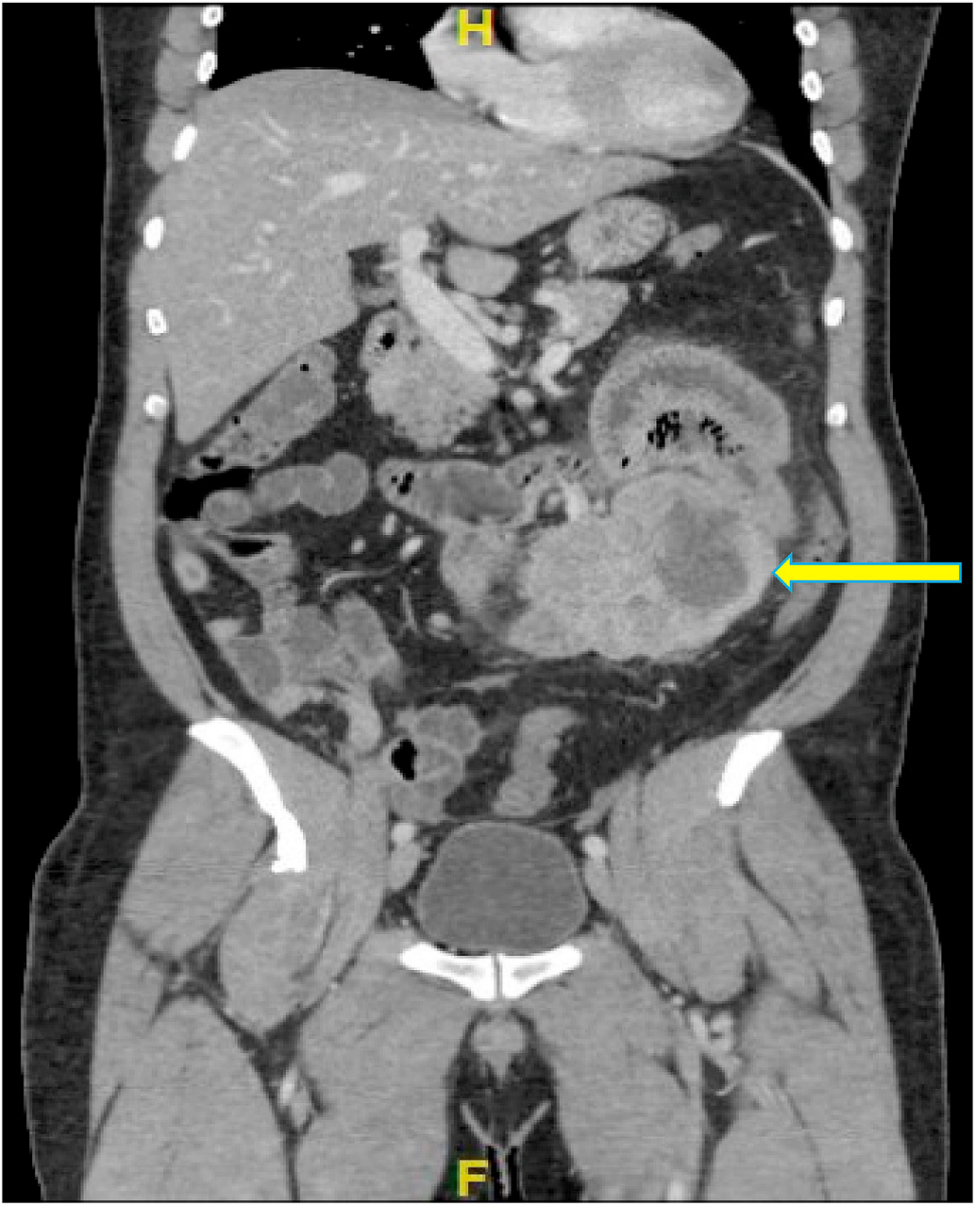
Coronal view of abdomen/pelvis CT scan showing the soft tissue mass, heterogeneous due to central necrosis. Proximal bowel dilation is seen due to the mass effect. The 3rd portion of the duodenum (horizontal portion) is seen dilated due to the mass. Compression of the adjacent bowel loops also is seen to cause a partial obstruction. Arrow pointing to mass.
